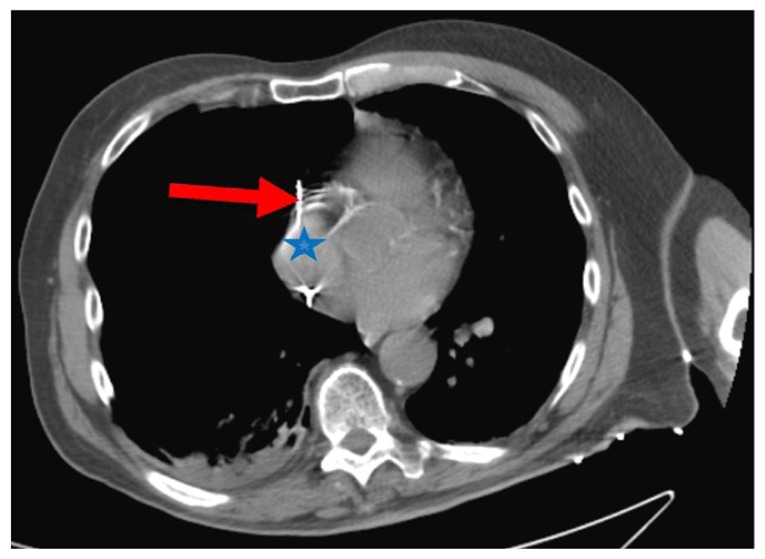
CT scan of the chest showing the tip of the pacemaker lead (red arrow) perforating through the right atrium (blue star).
